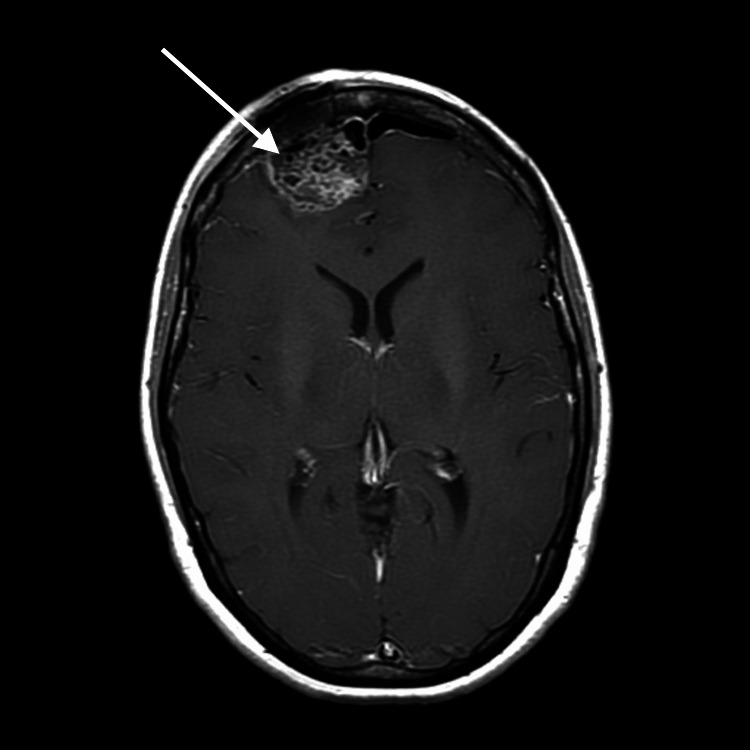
Post-contrast axial T1 MRI after craniotomy and resection of tumor (arrow) MRI: magnetic resonance imaging
